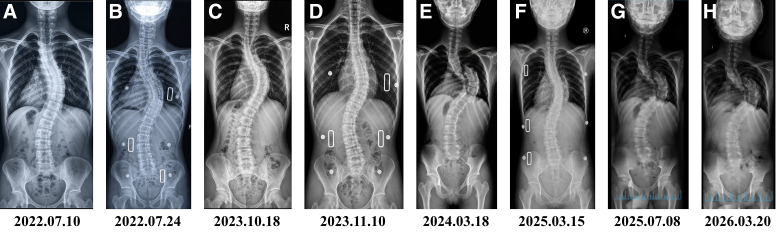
Standing, posteroanterior, whole-spine radiographs showing the progression and short-term follow-up of the spinal deformity. At initial presentation, the thoracic and lumbar Cobb angles were 48° and 26°, respectively. Before the start of the current treatment, the thoracic Cobb angle had progressed to 74° and the lumbar Cobb angle to 35°. The start date of the treatment was March 15, 2025. After 12 months of multimodal, conservative management consisting of a Chêneau brace, physiotherapeutic scoliosis-specific exercises, and adjunctive manual therapy, the thoracic and lumbar Cobb angles remained at 74° and 35°, respectively, with no further radiographic progression. Panels (A–H) indicate the time course from October 7, 2022, to March 3, 2026.
